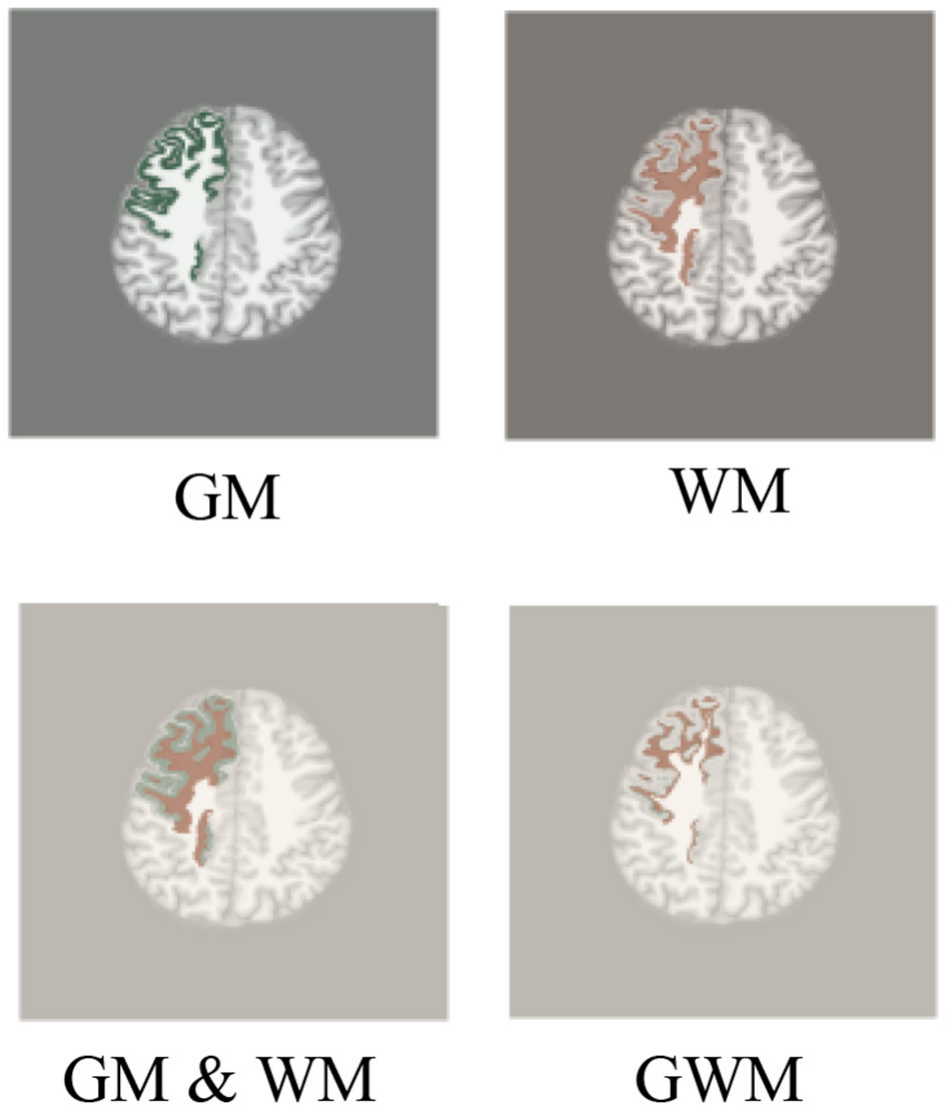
Region of interest of radiomics. We use T1 as background image to display region of interest, including GM, WM, GM & WM and GWM, similar to FLAIR and PET images.
